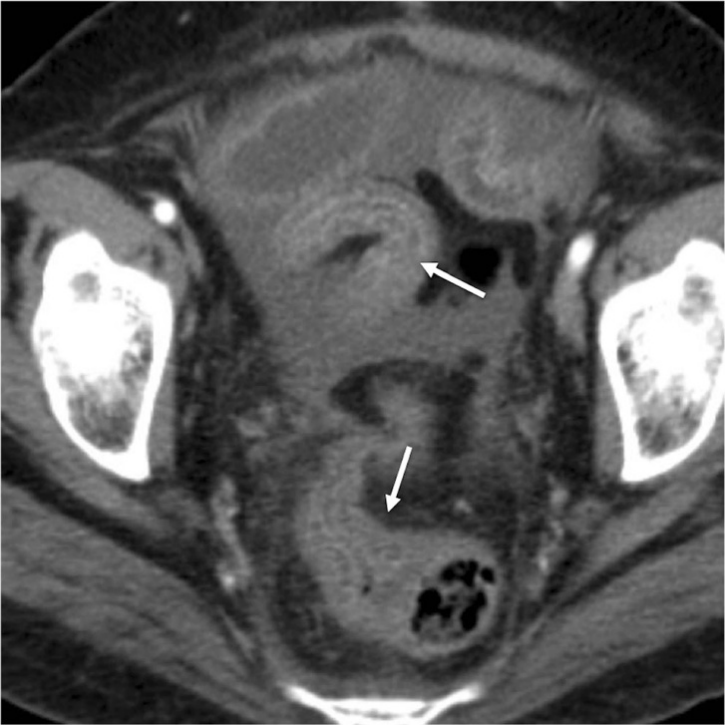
Radiation enteritis in a patient with cervical cancer. Axial contrast-enhanced CT scan shows concentric stratified wall thickening of low-lying small bowel loops (*arrows*). There are also some ascites. These findings in the context of radiation therapy are suggestive of radiation enteritis
